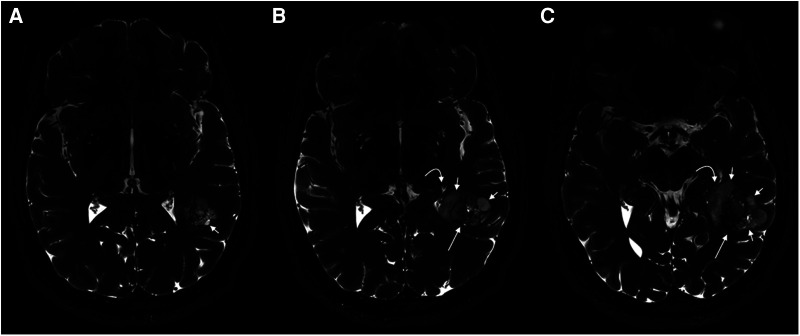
PLNTY. 7T MR imaging demonstrates internal characteristics of the mass on T_2_WI. From left to right (A–C), heterogeneous solid material is seen centrally (long straight arrows), while cystic components are located peripherally (short straight arrows). A mild associated mass effect is seen. This figure was originally posted by Benson et al. in *AJNR* (*American Journal of Neuroradiology*) in April 2020 (41).
